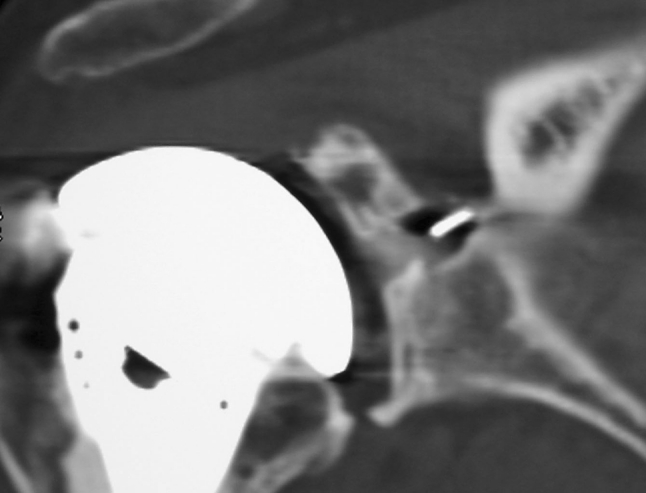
CT scan evaluation: peripheral bone integration of the central peg
